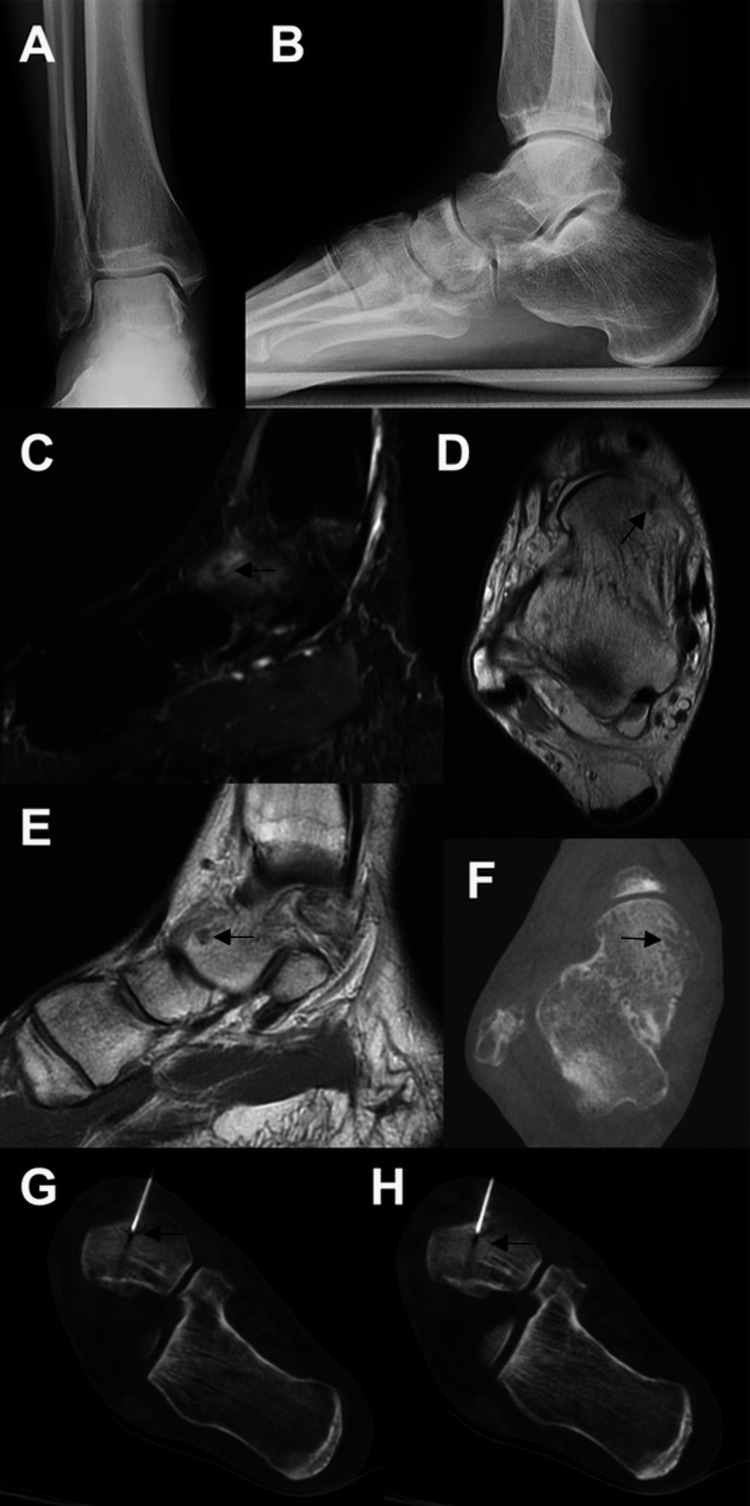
Images of the patient discussed in case one. Initial weight-bearing AP (A) and lateral radiographs (B) were unremarkable. The osteoid osteoma lesion is better delineated on the MRI; sagittal inversion recovery (C), axial proton density (D), and sagittal proton density (E) images are shown. The lesion was also seen on the weight-bearing axial CT (F). Intra-procedure CT images demonstrate successful targeting of the lesion with RFA (G, H). MRI: magnetic resonance imaging; CT: computed tomography; RFA: radio-frequency ablation
